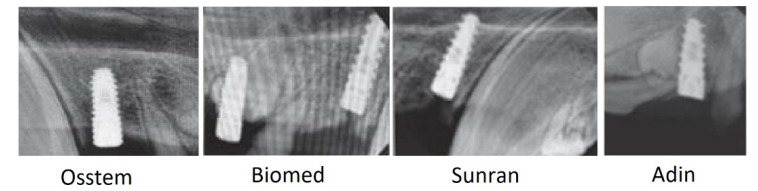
X-ray sampling of targeted radiography of the jaw bones of minipigs with different implantation systems one month after the installation of implants.
